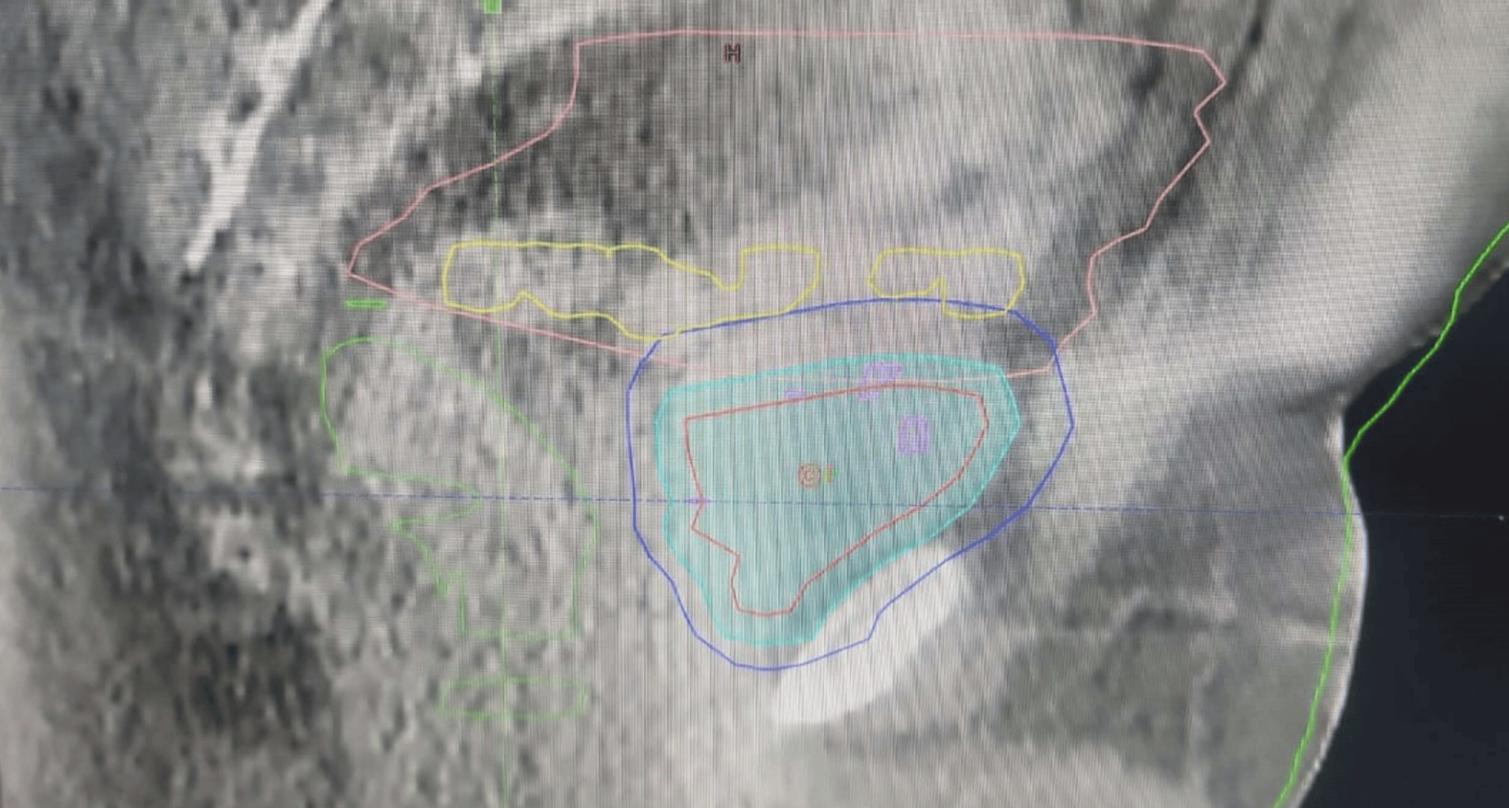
CBCT showing bladder shift beyond small PTV and within large PTV red: whole bladder; cyan blue: CTV; dark blue: PTV; pink: bowel bag; yellow: colon; green: rectum CBCT: cone beam computed tomography; PTV: planning treatment volume
